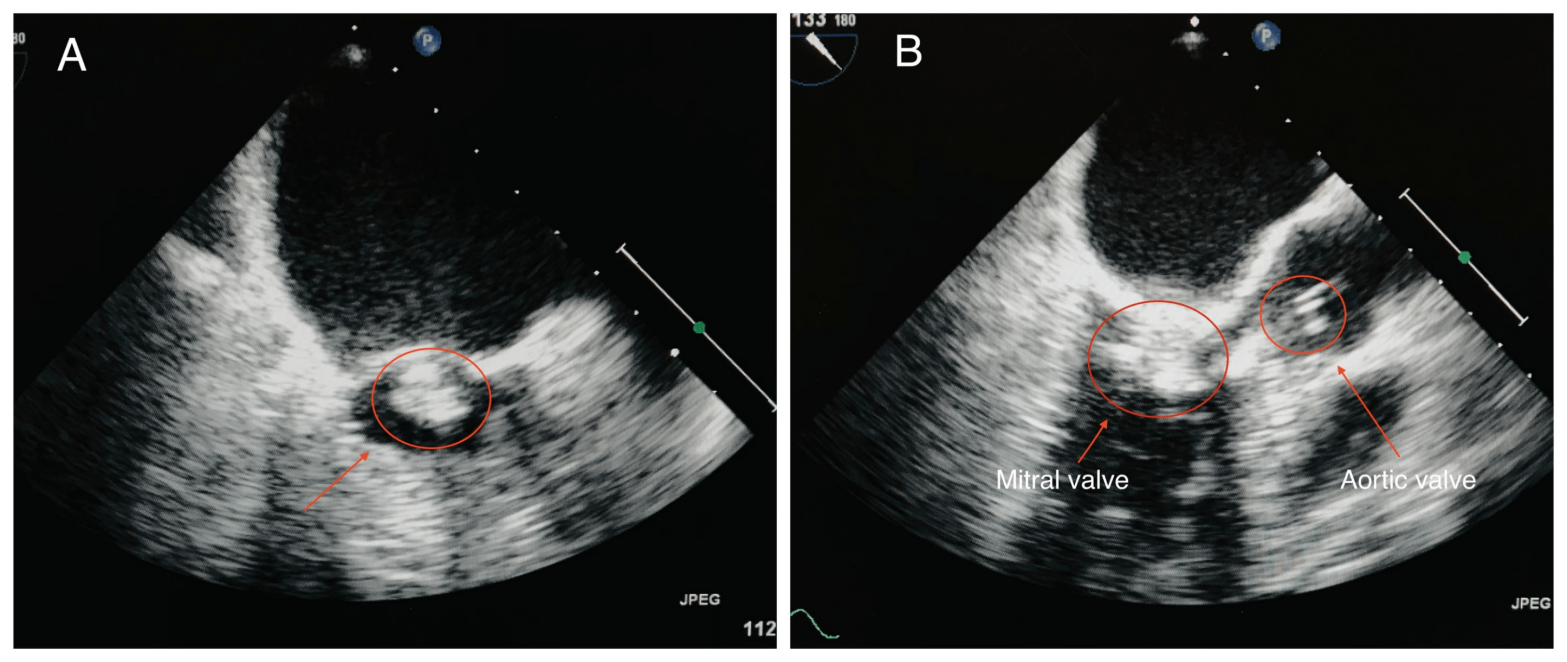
Transesophageal echocardiogram (A) revealing large echodensities attached to the anterior mitral valve leaflets and (B) echodensities on both the mitral and aortic valve leaflets
